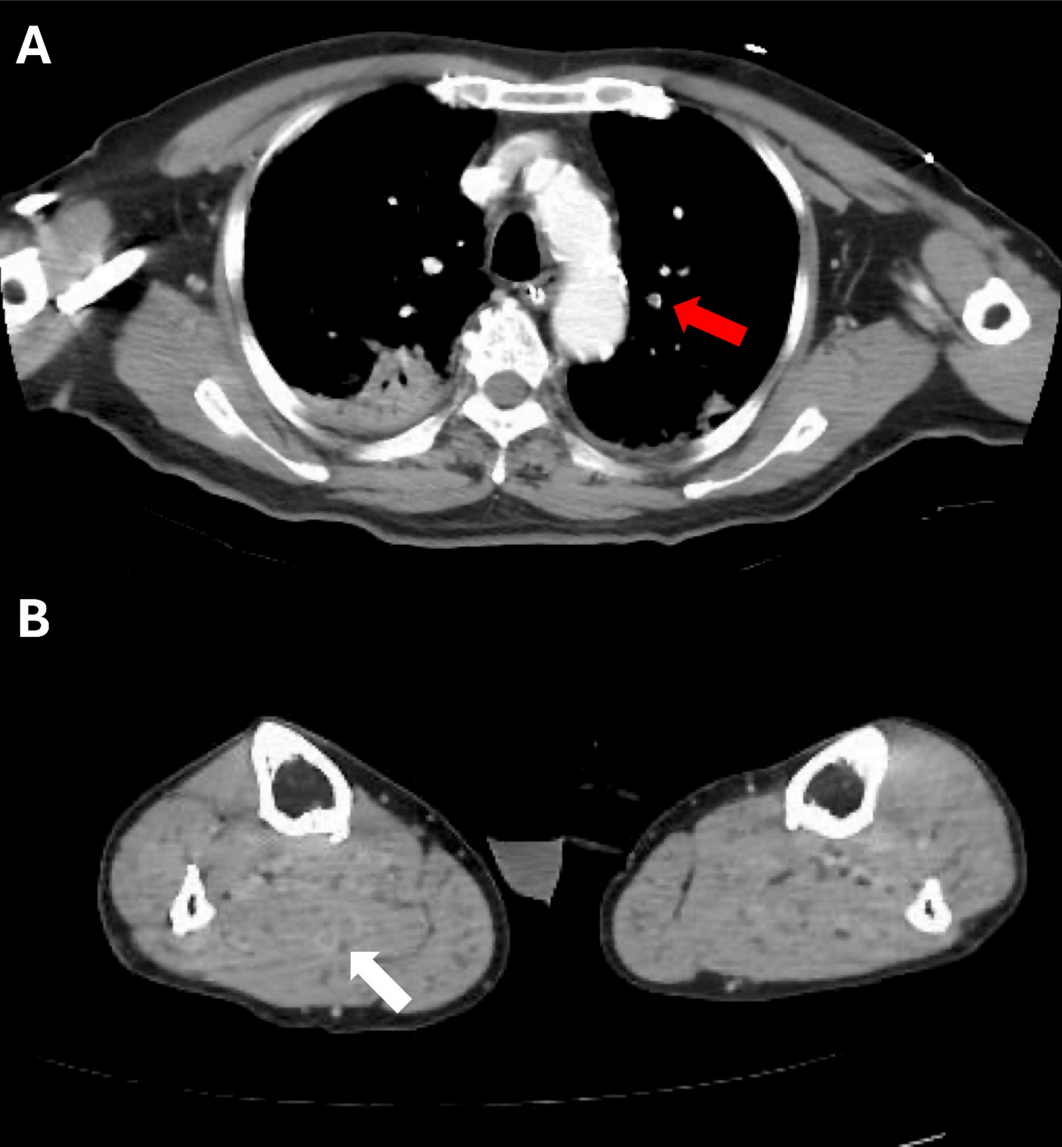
Contrast-enhanced CT on hospital day 10 The axial view of the contrast-enhanced CT scan on hospital day 10 revealed a small pulmonary thromboembolism (A, red arrow) and deep venous thrombosis in his right lower leg (B, white arrow).
